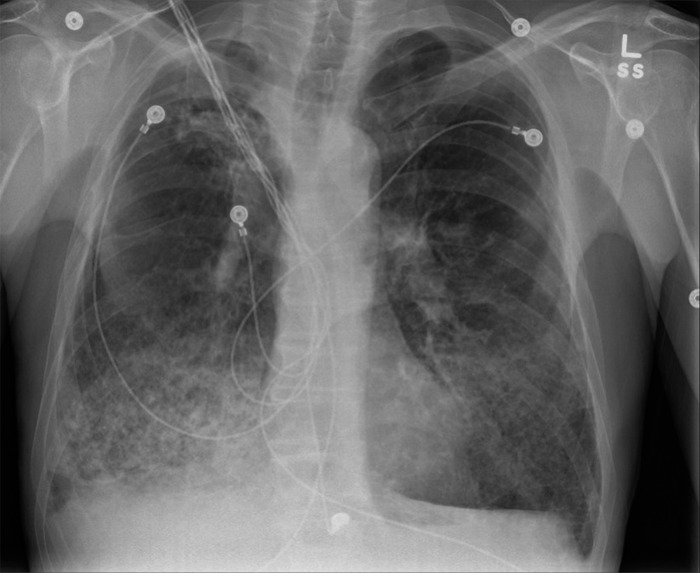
Admission chest x-ray shows bilateral infiltrates and chronic emphysematous changes.
